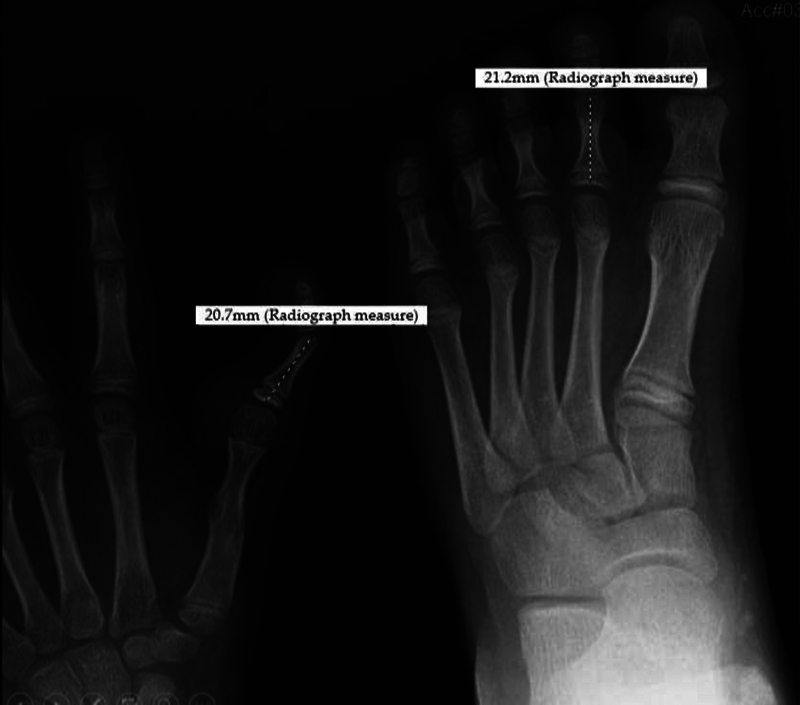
Radiograph of the right hand and foot, under the same magnification, with a comparative measure of the length of the proximal phalanx.
